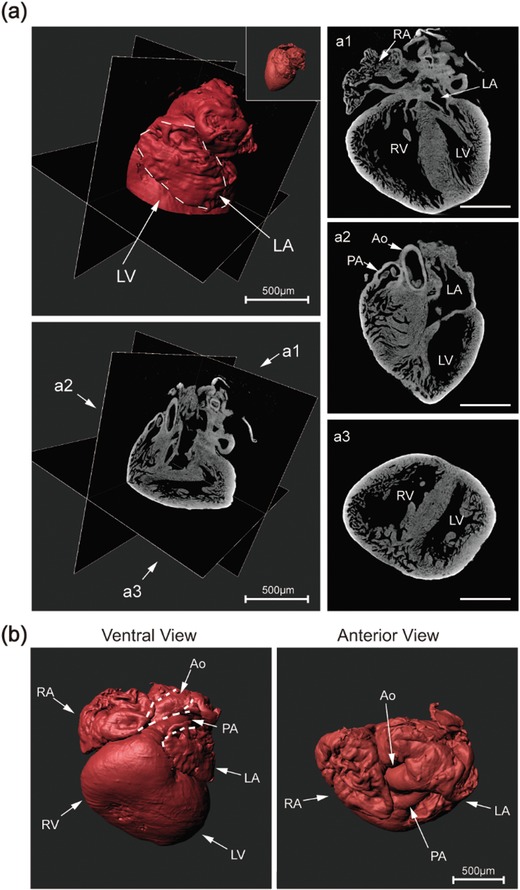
CT images cannot show 3D connections of cavities. a) 3D reconstruction data of cardiac CT images shows intracardiac structure in obtain plane sections at arbitrary angles. Right column indicate three sections of CT images at different angle. The insets show 3D myocardium image. RV, right ventricle; LV, left ventricle; RA, right atrium; LA, left atrium.; Ao, aorta; PA, pulmonary artery. Scale bar: 500 µm. b) 3D reconstruction of cardiac CT images shows outward appearance of ventricles, atrium, and great vessels.
